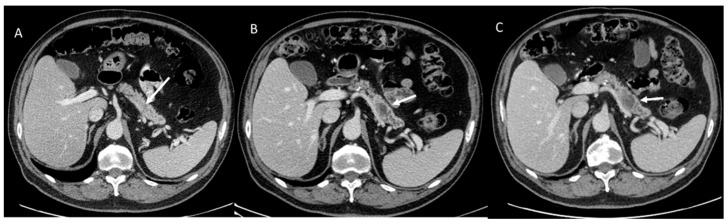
CT evaluation in melanoma patient with FAMM. In (A), arrow shows non-detectable lesion. In (B) arrow shows PDAC after 1 years (respect to CT assessment of (A)) and in (C) after 3 months (respect to CT assessment of (B)).
